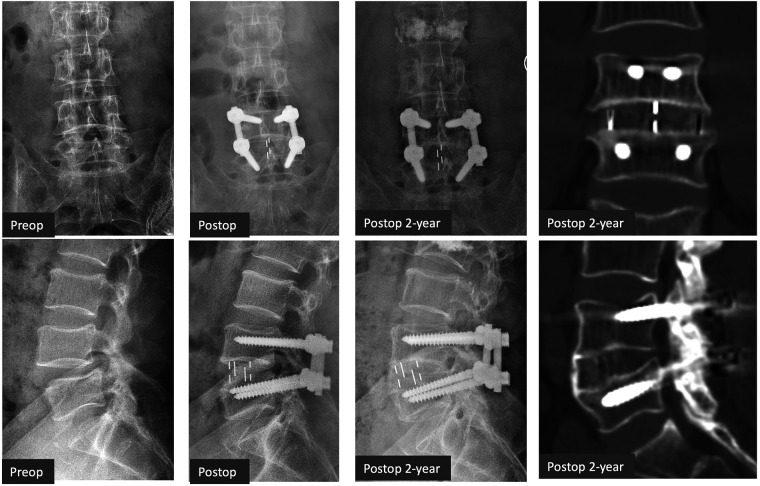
Case example of OLIF. A 63-year-old male patient with L4/5 degenerative spondylolisthesis had symptomatic low back pain and radiculopathy (ODI 35.6%, VAS-Leg 6, VAS-Back 6), which were relieved after OLIF with posterior laminotomy and fixation (ODI 10%, VAS-Leg 1, VAS-Back 2) at 2 years. Grade 1 fusion (apparent bone bridging) on CT scans was achieved at 2 years. OLIF, oblique lumbar interbody fusion; ODI, Oswestry disability index; VAS, visual analog scale.
